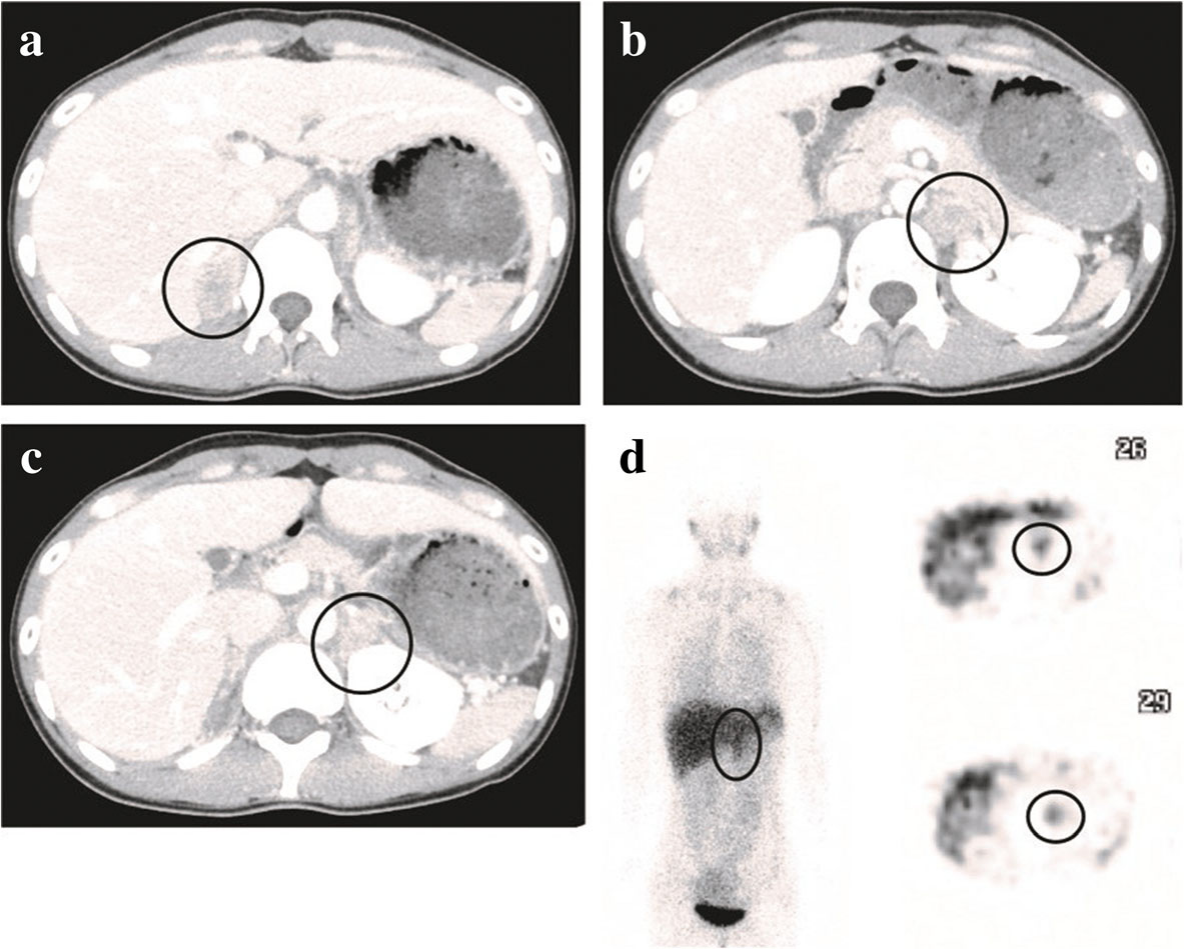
Contrast-enhanced CT showed enhanced masses in the right adrenal gland (a), left adrenal gland (b), and left renal hilus (c). d
